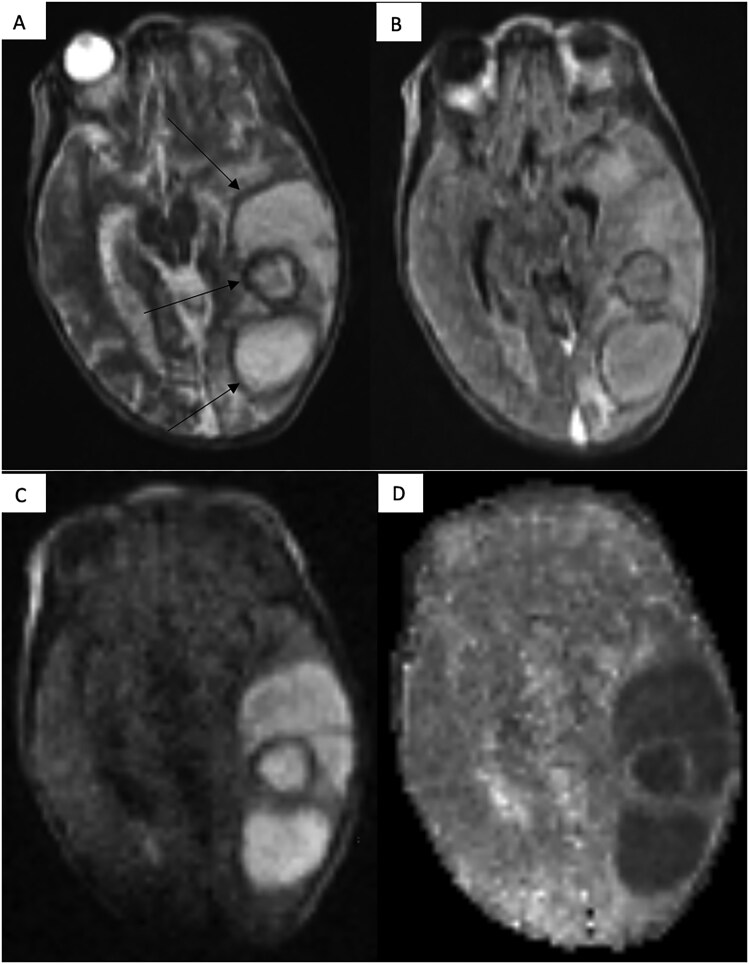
Complex, multicystic left temporal lobe abscess (indicated by arrows) with relatively mild surrounding panrechymal oedema and localised mass effect on the axial T2W sequence in Panel A. Panel B demonstrates the hypointense thick capsule on the axial FLAIR sequence and restricted diffusion within the lesion consistent with pus on the axial DWI 900 sequence and the corresponding ADC map in Panels C and D.
